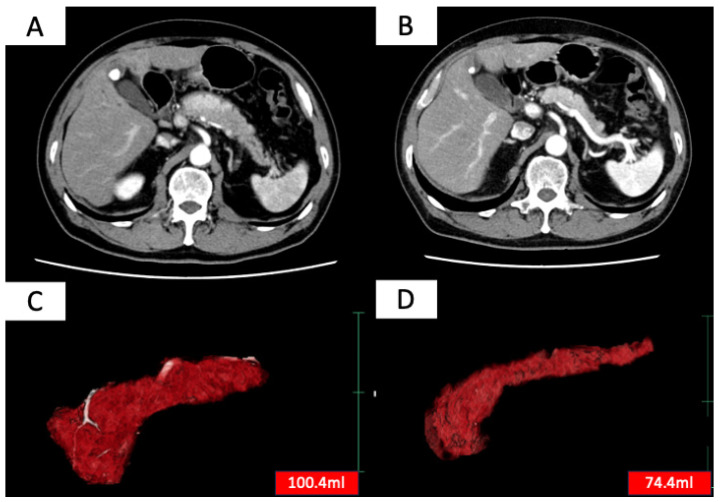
Contrast-enhanced CT images before (A) and after (B) steroid treatment, and whole pancreas images before (C) and after (D) treatment extracted using SYNAPSE VINCENT (Fujifilm, Japan). SYNAPSE VINCENT allows not only visual evaluation of the pancreas but also the measurement of pancreatic volume.
